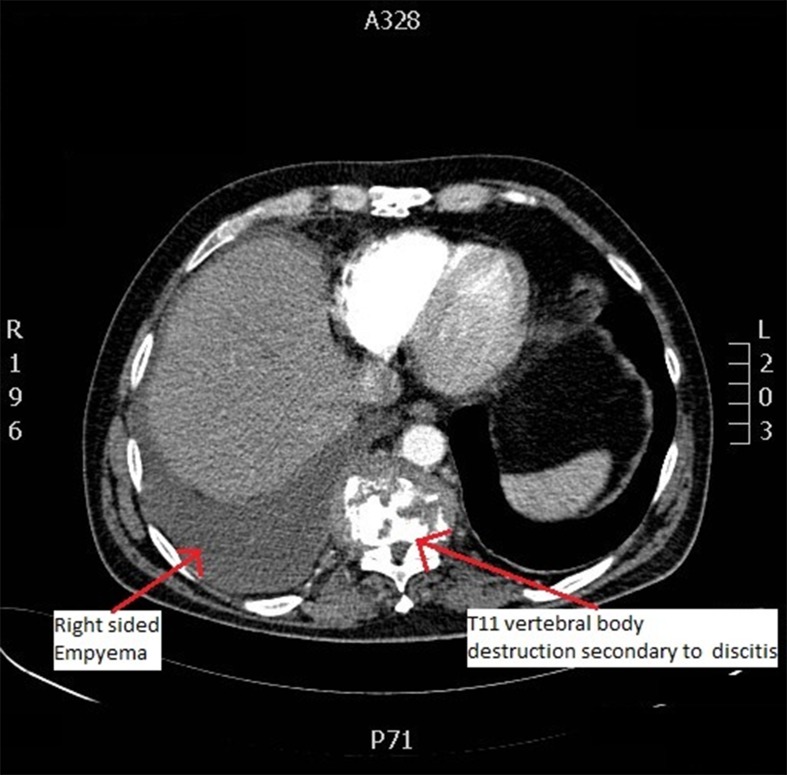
Axial computed tomography of the thorax showing right-sided empyema and destruction of T11 vertebral body due to discitis and paraspinal abscess
